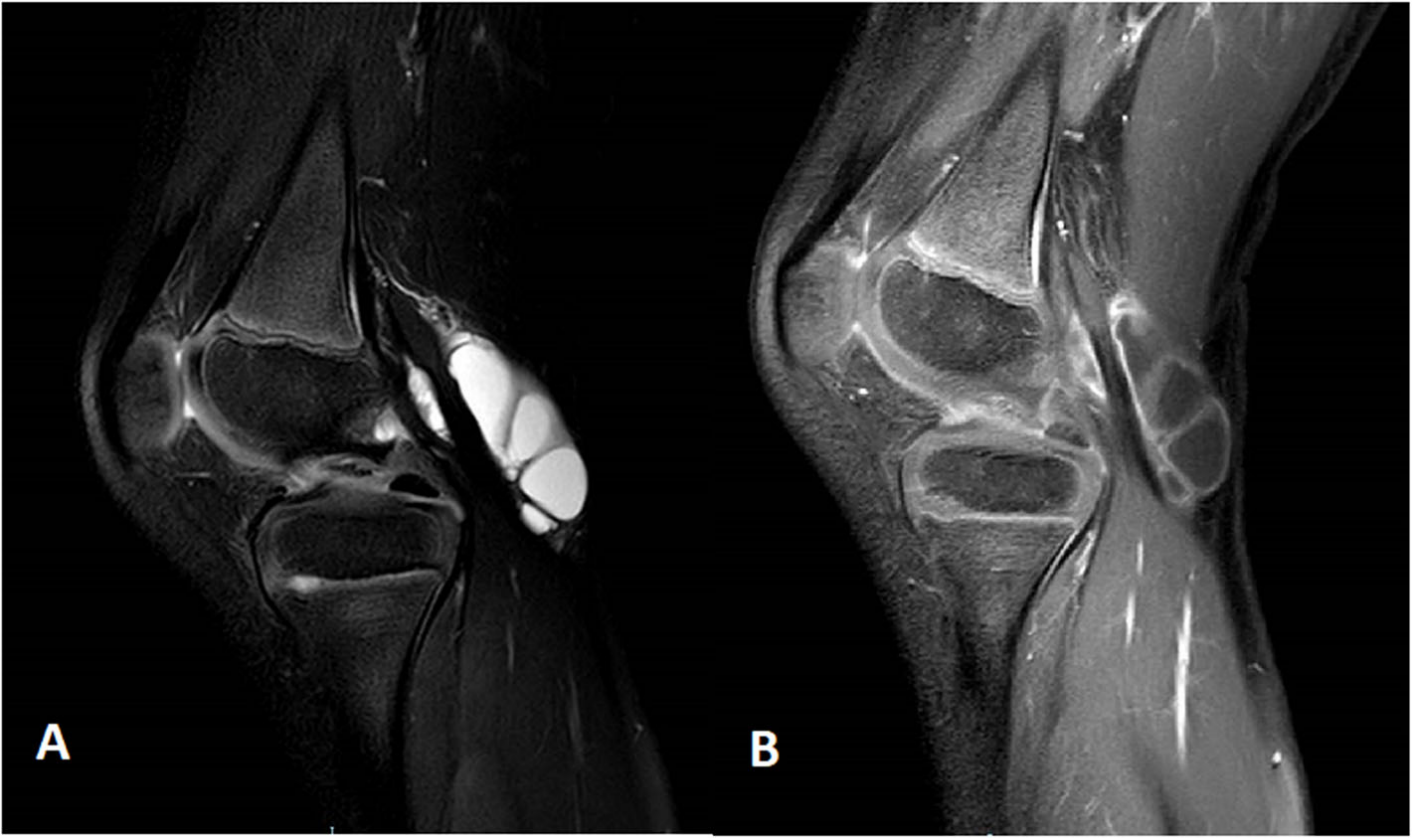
Magnetic Resonance Imaging (MRI). MRI sagittal view of the left knee: T2-weighted image (A), and T1-weighted contrast-enhanced image (B) showing the presence of a voluminous multiloculated Baker’s cyst
